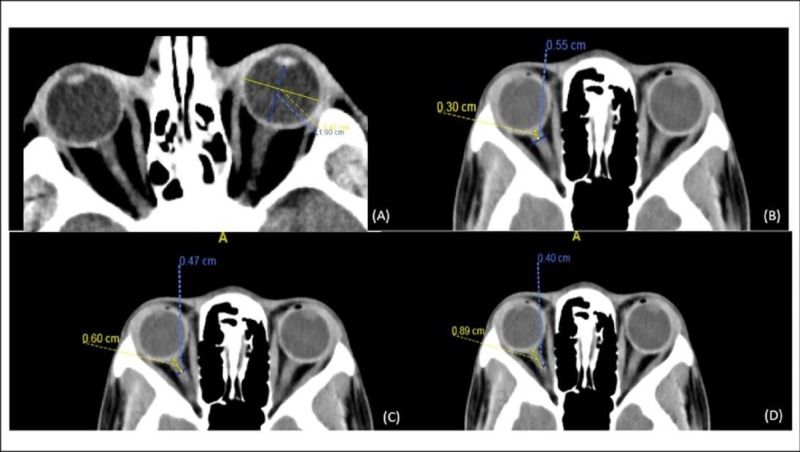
Optic Nerve Sheath Diameter (ONSD) and Eyeball Transverse Diameter (ETD) Measurement A) Eyeball transverse diameter measurement technique on computed tomography: Yellow line for ETD measurement is the largest line through the eyeball drawn perpendicular to the axis of the ONSD (blue line); B) shows a measurement of ONSD at 3 mm distance, (C) shows ONSD at 6 mm distance, and (D) shows the ONSD at 9 mm distance.
